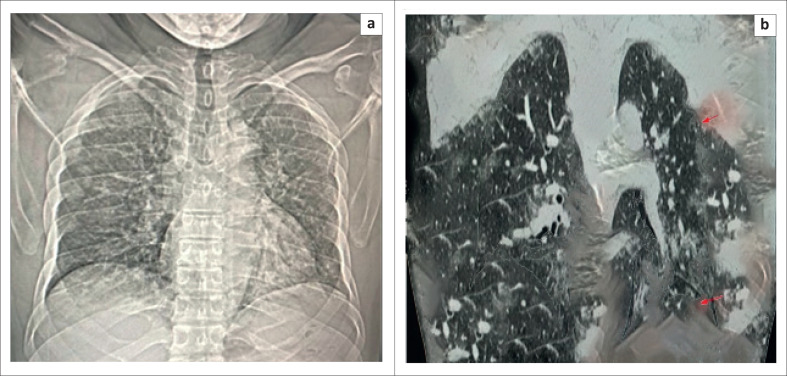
Radiological images of a 55 year old male patient with COVID-19 (37 Military Hospital, Accra, Ghana, May 2020). Case 3: (a) Postero-anterior chest x-ray showing bilateral ground-glass opacification and right upper and middle zone peripheral patchy opacities. (b) High resolution computerised tomography scan, coronal view, showing bilateral, ground-glass opacities with thickened interlobular and intralobular lines with ‘crazy paving’ appearance. Bilateral peripheral and subpleural opacities (red arrows).
